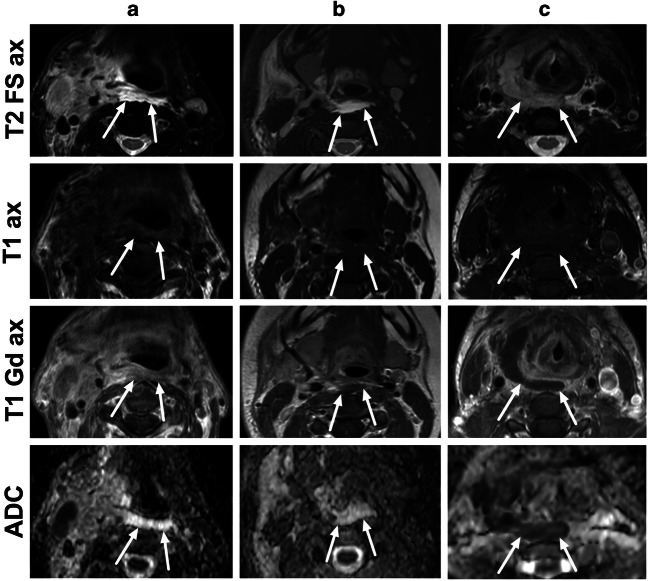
Different types of RPE in axial fat-suppressed T2-weighted Dixon images (first row), axial pre-contrast T1-weighted images (second row), axial post-contrast in-phase Dixon T1-weighted images (third row), and axial ADC maps (fourth row), from three patients (a–c). a A 63-year-old male with tonsillitis had high T2 signal, contrast enhancement on T1, and no purulence on ADC (enhancement-type RPE). b A 23-year-old female with parotitis had high T2-signal, non-enhancing fluid on T1, and no purulence on ADC (fluid-type RPE). c A 41-year-old female with a throat infection had an intermediate T2-signal, non-enhancing fluid on T1, and low ADC consistent with purulent fluid (true retropharyngeal abscess)
